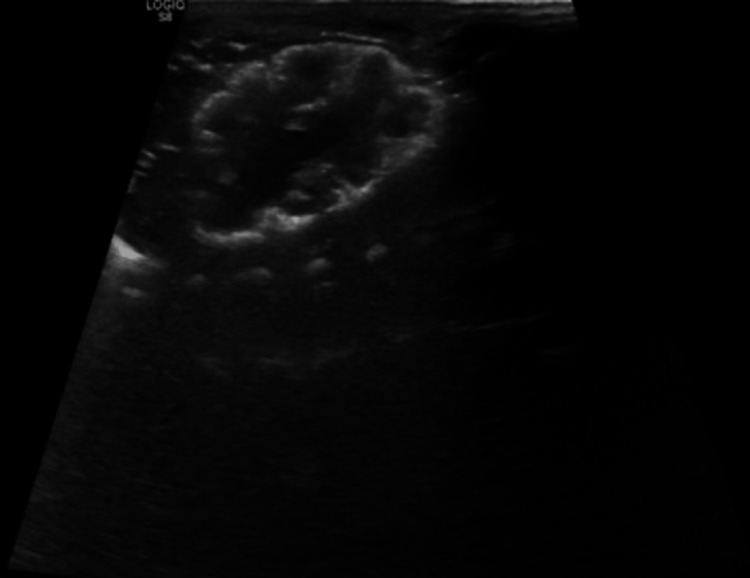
Abdominal ultrasound of right kidney showing loss of corticomedullary differentiation with minimal perinephric collection.
